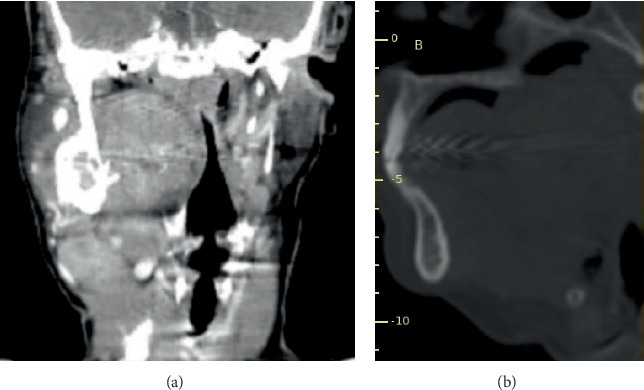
Severe airway obstruction shown on (a) computed tomography coronal plan and (b) sagittal cut.
